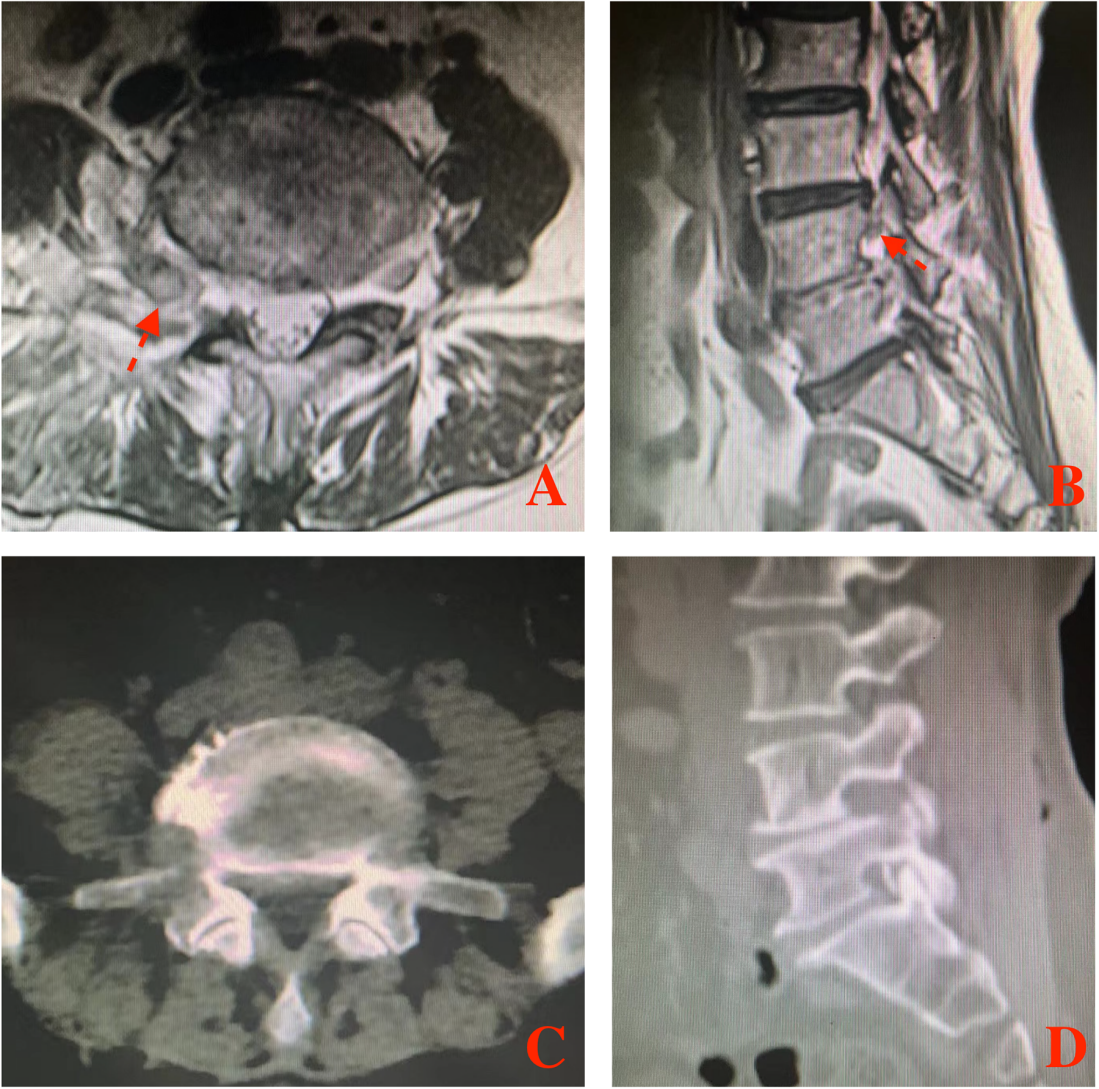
The extraforaminal area was shown in postoperative MRI and CT. (A) MRI axial views of L4–5; (B) MRI sagittal view of L4–5; (C) CT axial views of L4–5; (D) CT sagittal view of L4–5. Red arrow indicated the L4 exiting nerve root.
